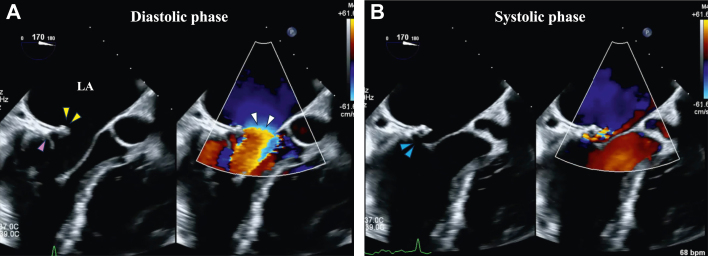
Transesophageal Echocardiography Findings Mid esophageal long axis view in diastolic phase (A) and systolic phase (B). Yellow arrowheads indicate a prosthetic valve ring, pink arrowheads indicate the original mitral valve annulus, white arrowheads indicate accelerated flow, and blue arrowheads indicate acoustic shadow. Abbreviation as in Figure 4.
